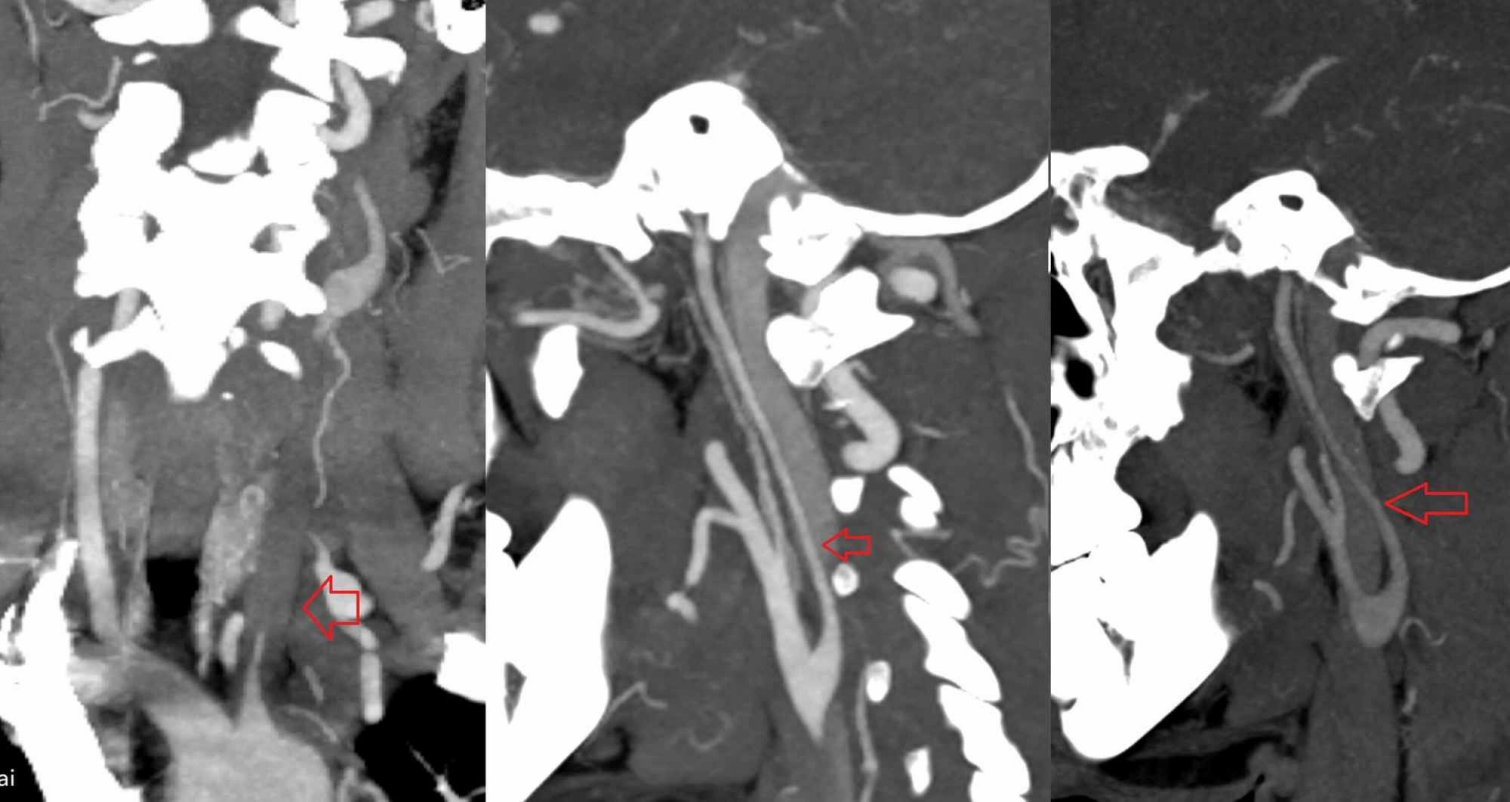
CT angiography of the left common carotid artery from aortic origin and left internal carotid artery Chronic occlusion of the left common carotid artery (right) and the chronic narrowing of the left internal carotid artery (middle and left).
